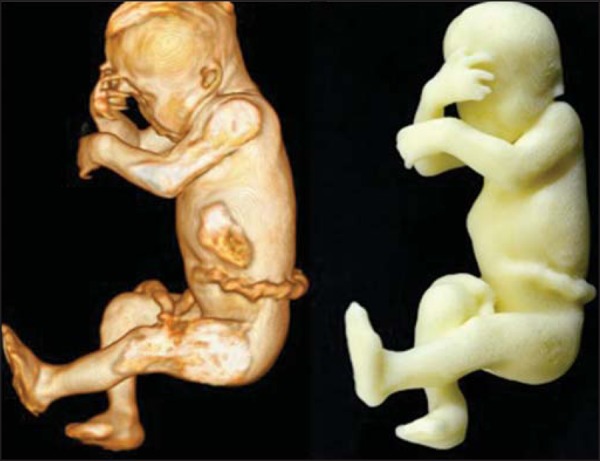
Whole-body 3D reconstruction from the MRI and physical model.
